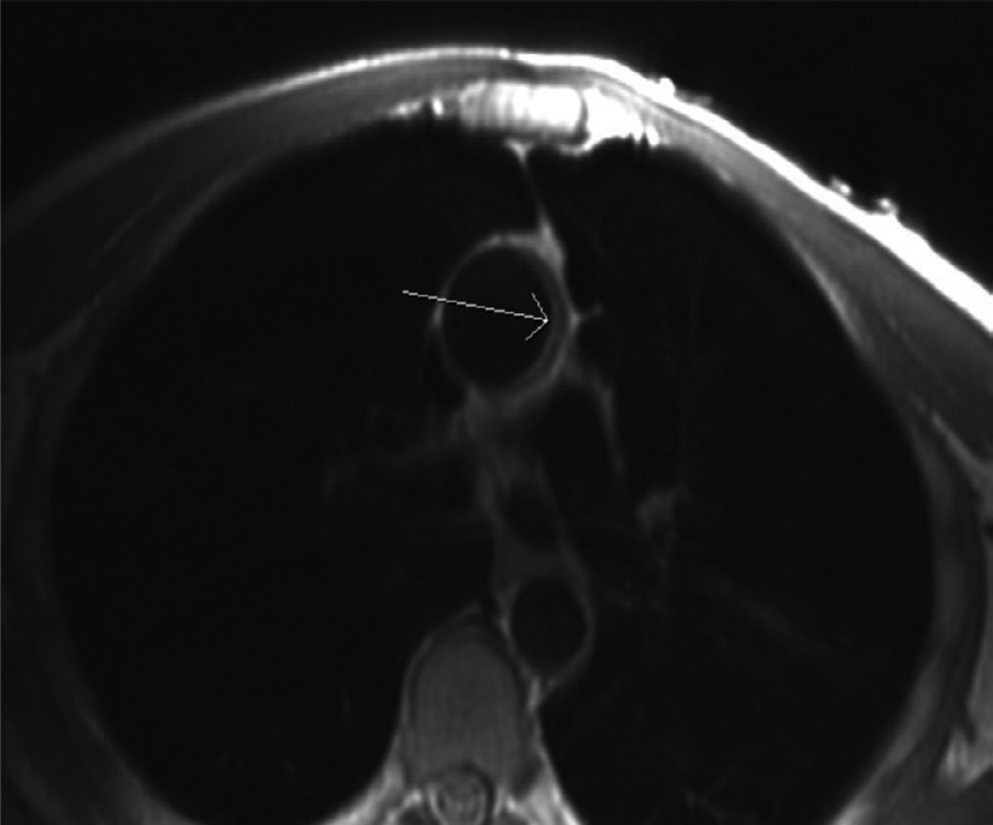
Cardiovascular MRI demonstrates significant atherosclerosis in ascending aorta (Patient # 2)
